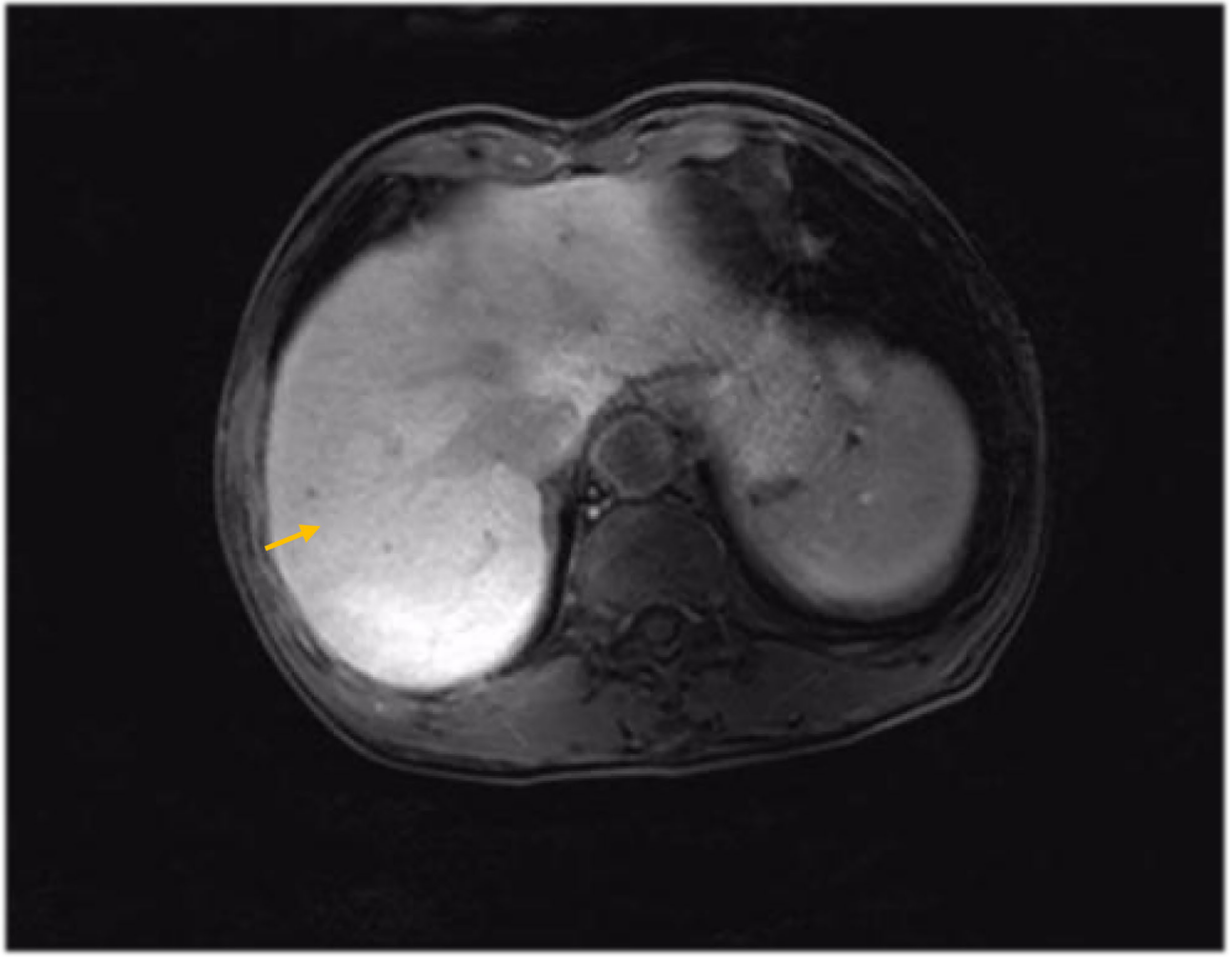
MRI findings during the patient’s last chemotherapy session (liver metastases disappeared).
